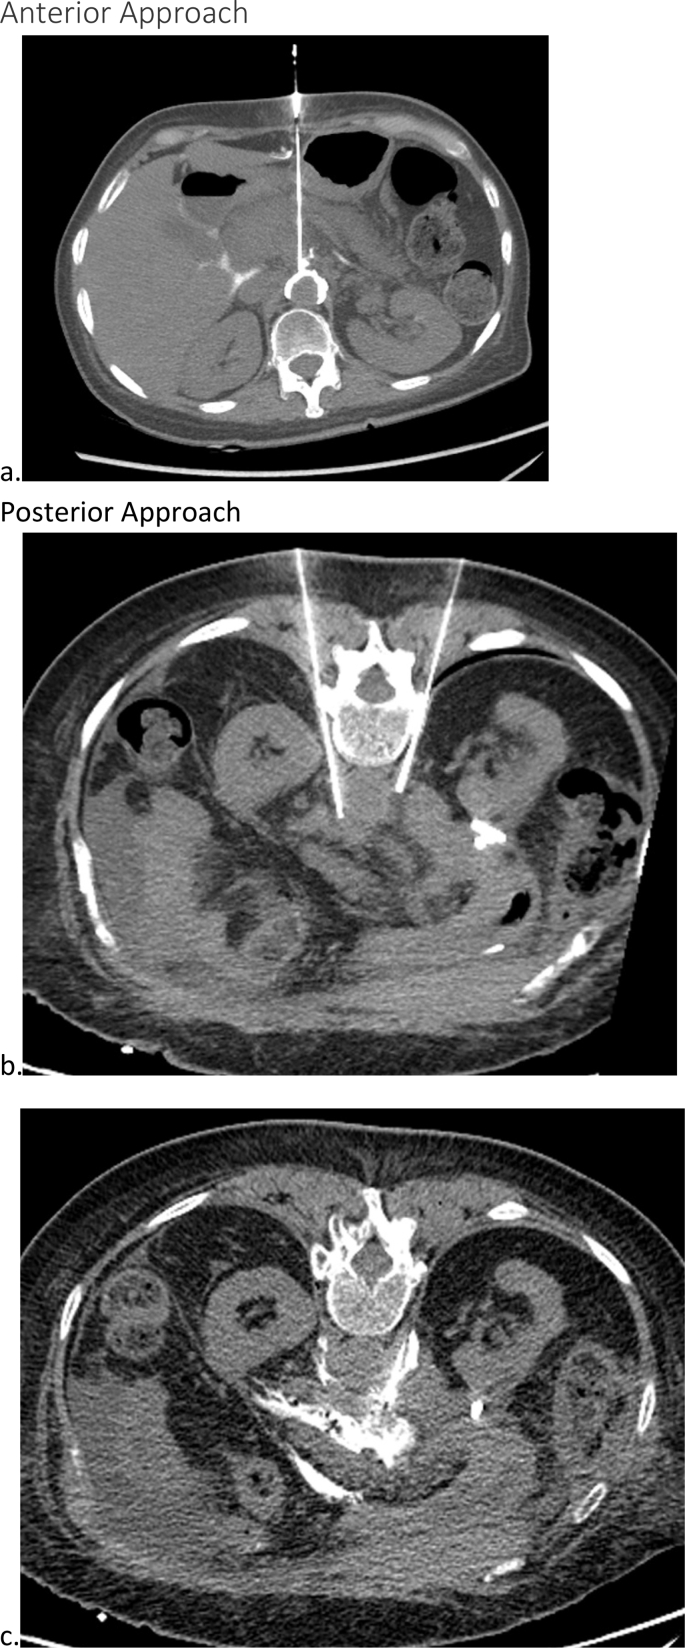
Celiac Block Technique Under CT Guidance (Anterior and Posterior approaches) Celiac plexus nerve block under CT guidance (anterior and posterior approach). CT guided transgastric approach to celiac axis using a 22 ​g needle, confirmed with dilute contrast injection distributing along the celiac axis and adjacent tissue (a). Transhepatic approaches may also be performed safely. CT guided posterior approach to celiac plexus, bilateral approach, using a paraspinal technique with confirmation of distribution via injection of dilute contrast (b+c). A single needle approach may be utilized, provided adequate distribution of contrast is achieved in the region of the celiac axis.
